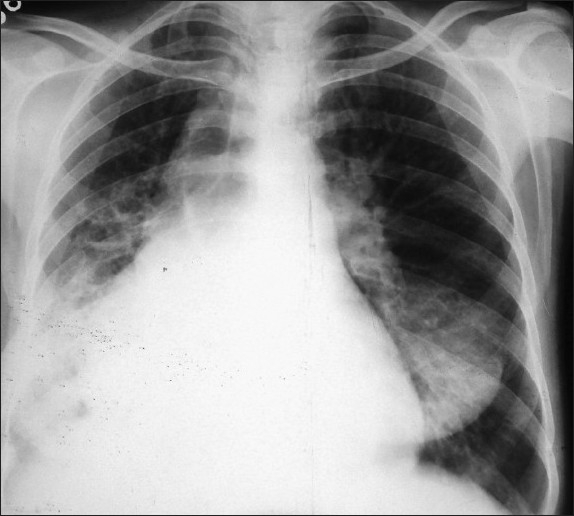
Chest roentgenogram showing dextrocardia.
